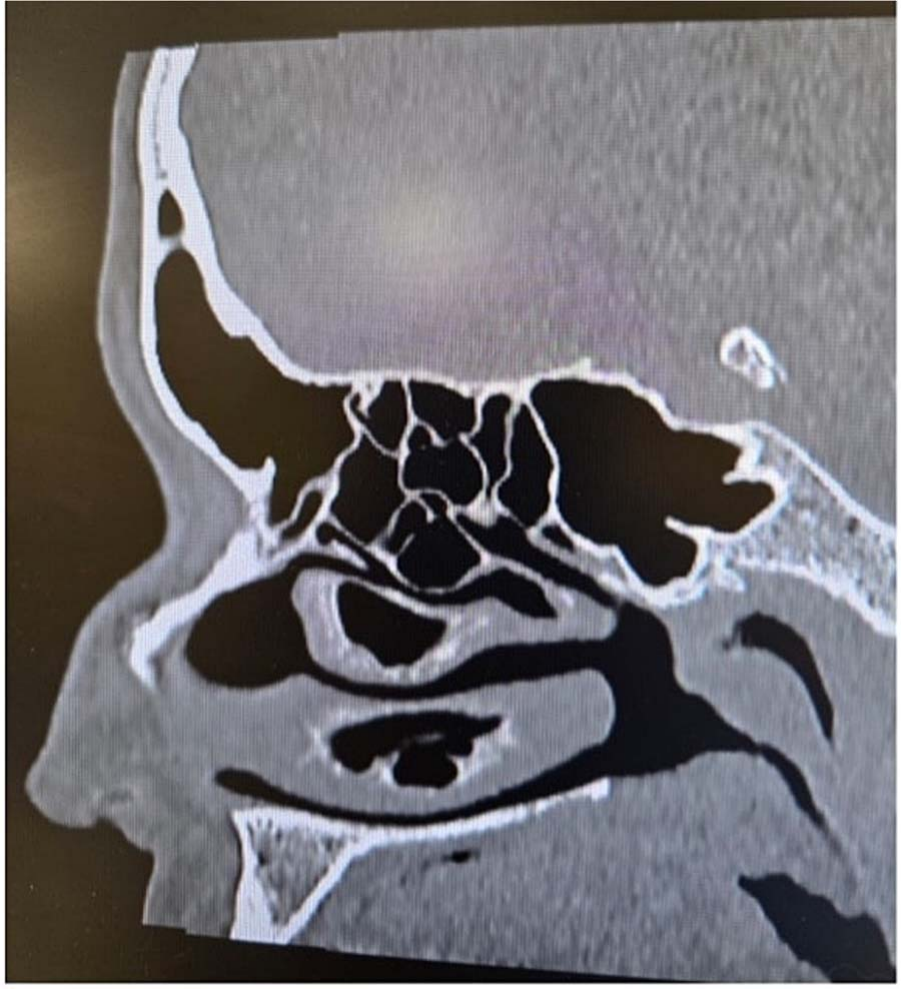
Paranasal sinus CT scan in the sagittal plane demonstrating middle concha bullosa and inferior concha bullosa.
